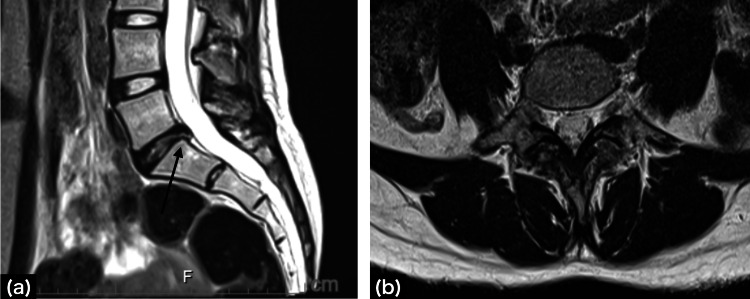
Six-month postoperative magnetic resonance T2-weighted image (a) Sagittal view; a posteriorly protruding disc at L5/S1 height is observed. The white arrows indicate mild HIZs. (b) Axial view of L5/S1; no obvious disc herniation is observed. HIZs: high-intensity zones
